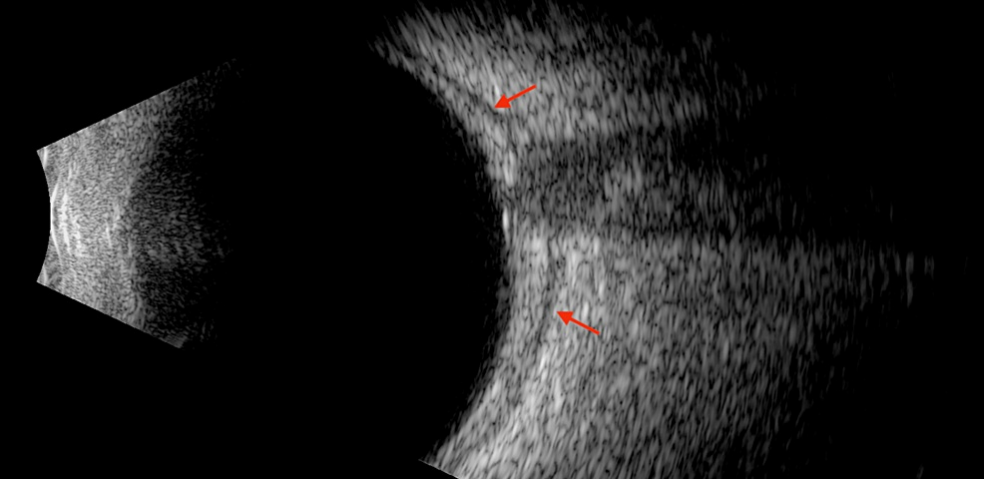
Ophthalmic ultrasound (B-scan) of the right eye. Positive T-sign (red arrows).
